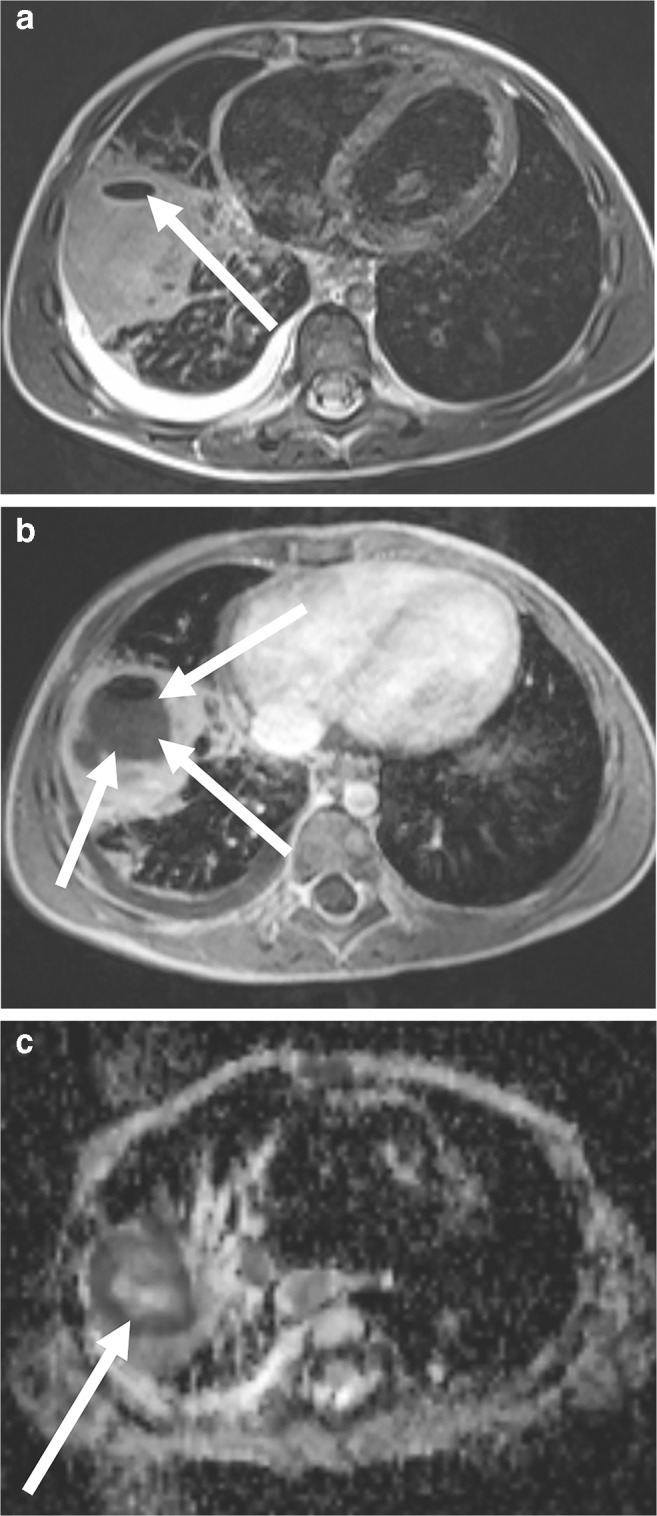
Lobar pneumonia with an abscess on lung MR in a 3-year-old girl (MR parameters in Tables 2 and 3). a On axial T2-weighted turbo spin-echo image, the abscess is not clearly distinguishable from the surrounding inflammatory tissue. It only demarcates itself by ventral air accumulation (*arrow*). b In axial T1-W gradient echo sequence after contrast medium administration, the abscess (*arrows*) is clearly distinguishable from the inflammatory area. Note that the administration of a contrast agent for lung examinations is almost only necessary for this indication. c Apparent diffusion coefficient (ADC) map from the axial diffusion-weighted imaging sequence: The low ADC values (*arrow*) prove the purulent content within the cave
